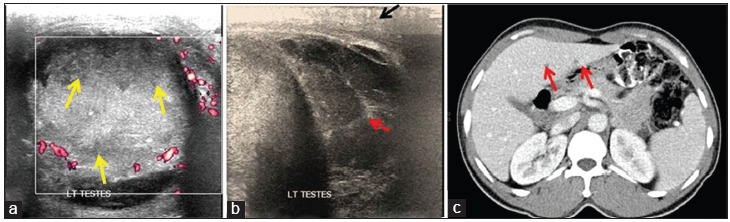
A 43-year-old male with complicated testicular tuberculosis presenting with scrotal pain and swelling. (a) Color Doppler sonographic image demonstrates diffuse, ill-defined heterogeneous hypoechoic area with increased peripheral vascularity but lack of central vascularity (yellow arrows). (b) Gray scale ultrasound image showing scrotal wall edema (black arrow) and multiseptated hydrocele and debris (red arrow). (c) Contrast-enhanced axial computed tomography image of abdomen in same patient shows hypoechoic lesions in the left lobe of liver (red arrows) likely representing tubercular granulomas.
